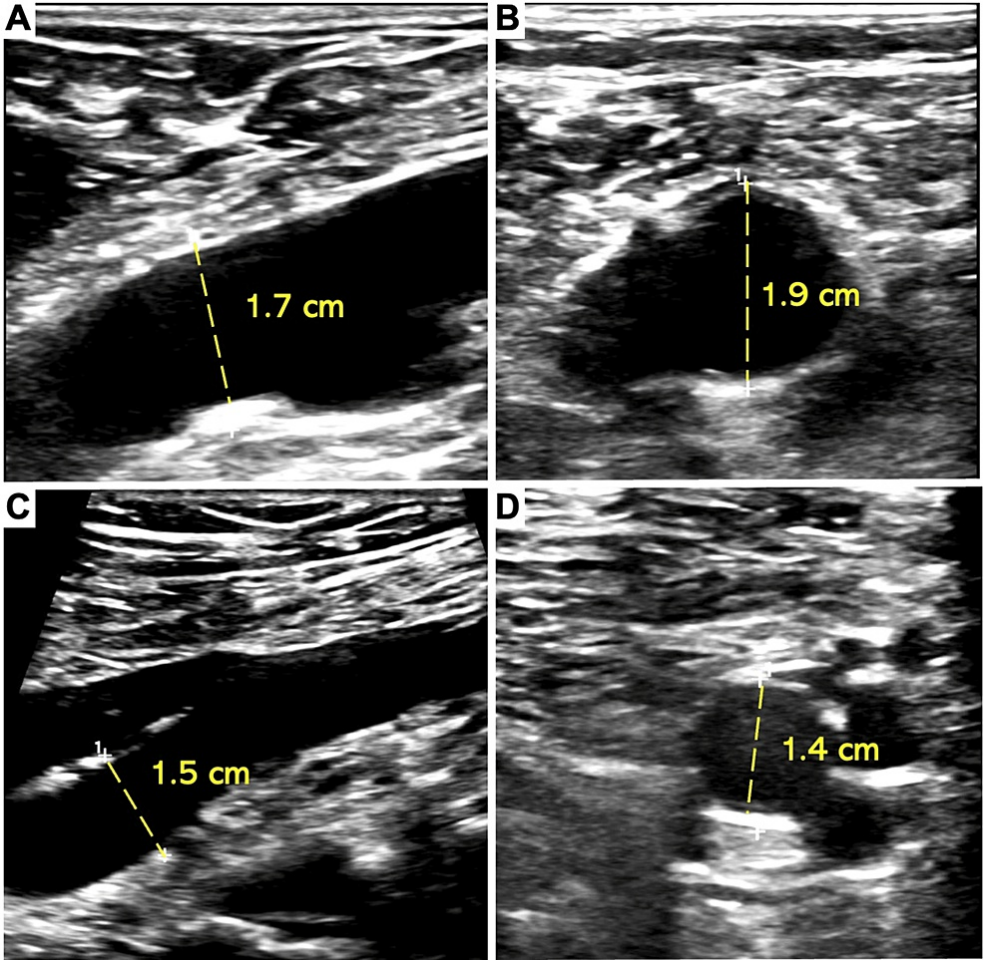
Arterial ultrasound of the bilateral lower limbs. Aneurysms of the (A) right common femoral artery (1.7 cm), (B) left common femoral artery (1.9 cm), (C) right popliteal artery (1.5 cm), and (D) left popliteal artery (1.4 cm).
